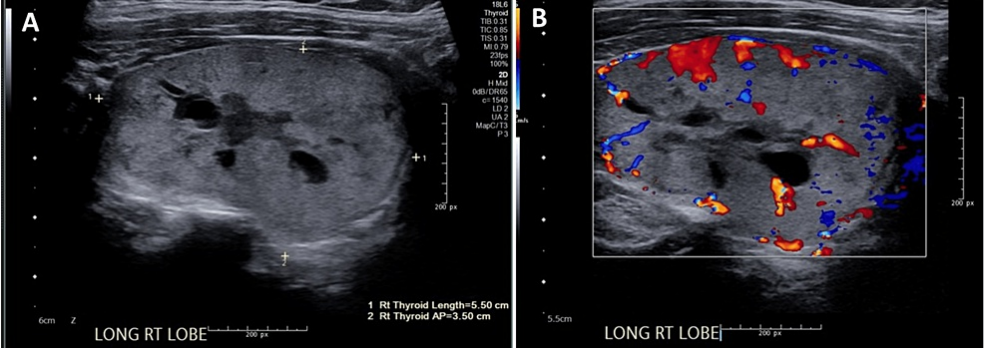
(A) Ultrasound and (B) color duplex of the right thyroid shows 5.1 x 2.9 x 3.5 cm biopsy proven TIRADS category 2 right thyroid nodule.
